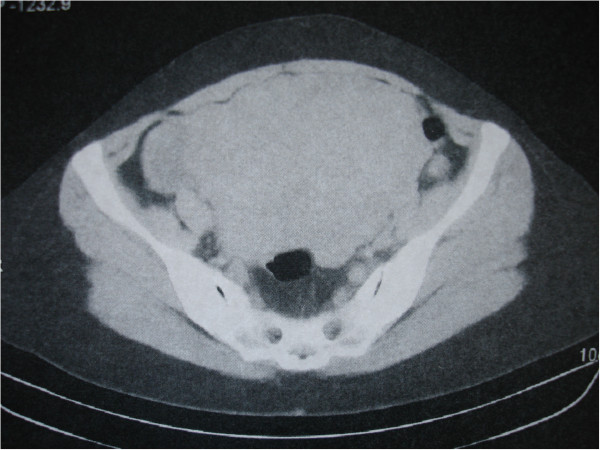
Computed tomography image. Large mass occupying the lower abdomen.
